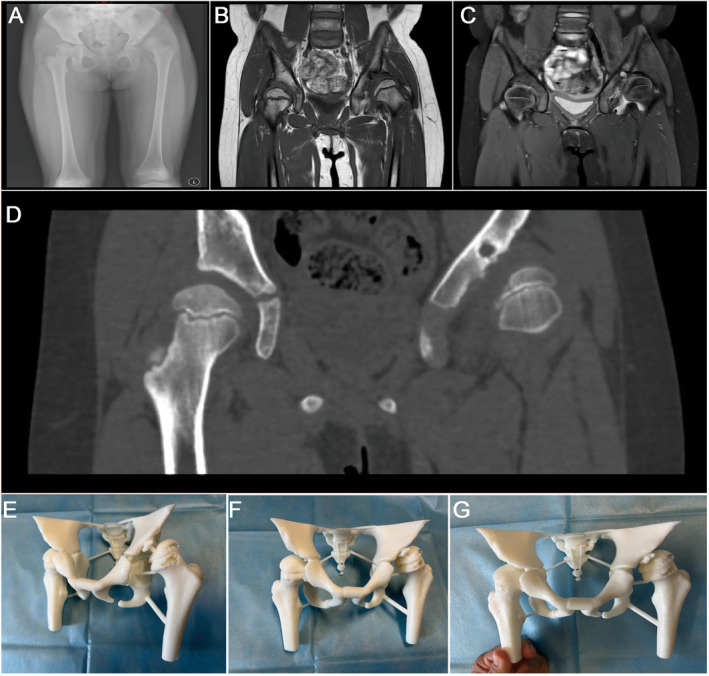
Preoperative imaging and 3D‐printed models. (A) Preoperative anteroposterior radiographs of the pelvis; magnetic resonance imaging of the osteocystic lesion (B, C); computed tomography of the hip joint osteocytic lesion (D); and 3D‐printed one‐to‐one models (E–G)
